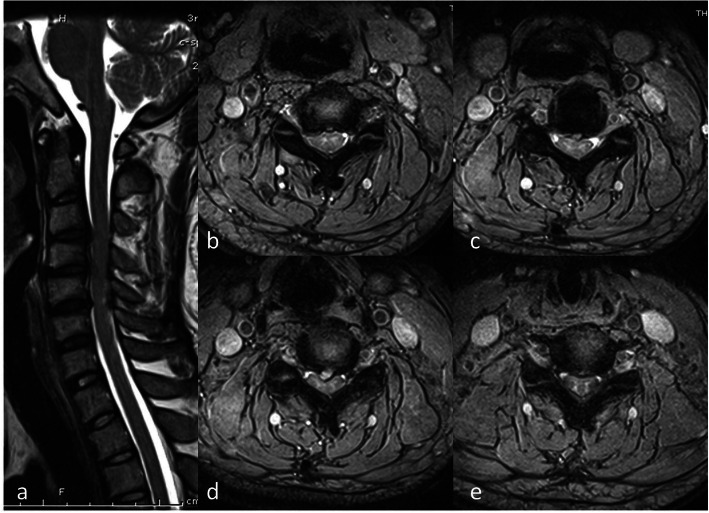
a A 56-year-old male patient was diagnosed as MCSM with typical symptoms of superior motor neurons compression. T2-weighted sagittal MRI showed spinal cord compression in C3/4, C4/5, C5/6. b–e C3/4, C4/5, C4/5, and C5/6 spinal cord compression on T2-weighted axial MRI
